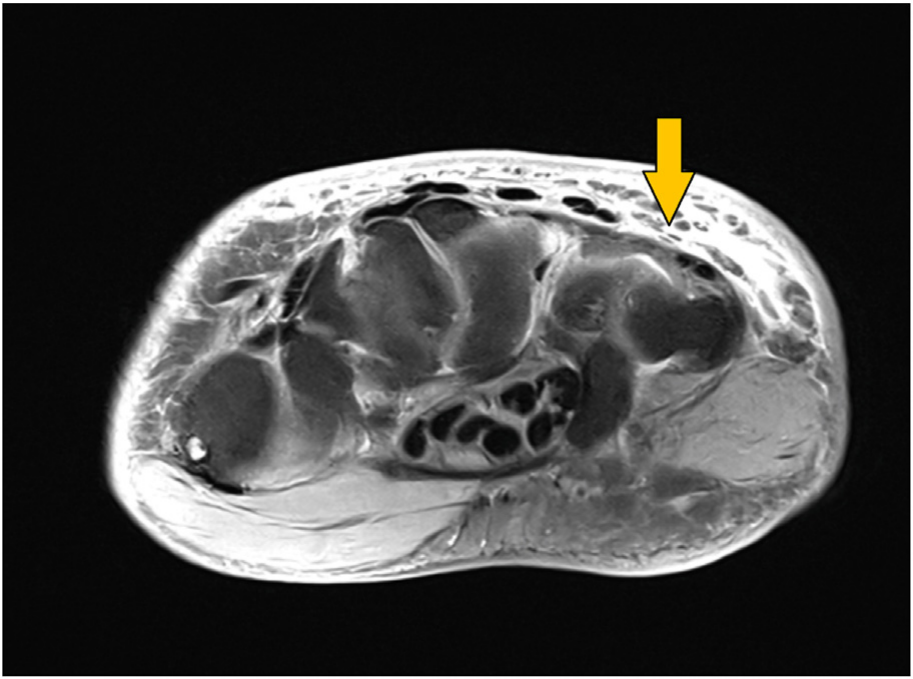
Magnetic resonance imaging of the right hand during treatment (T2 coronal view): *yellow arrow* pointing to the subcutaneous mucinosis.
